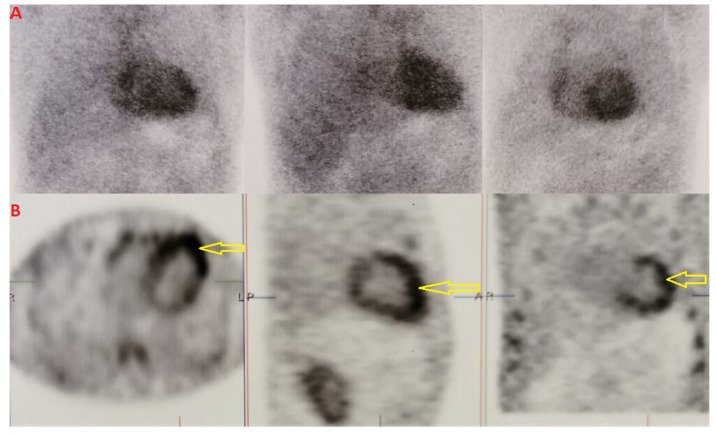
Bone scintigraphy with ^99m^Tc-DPD and single-photon emission computerized tomography (SPECT) imaging. (A) Bone scintigraphy with positive result defined as grade 3 = high myocardial uptake, greater than the bone. (B) SPECT confirming intra-myocardial uptake in both right and left ventricles (yellow arrows).
